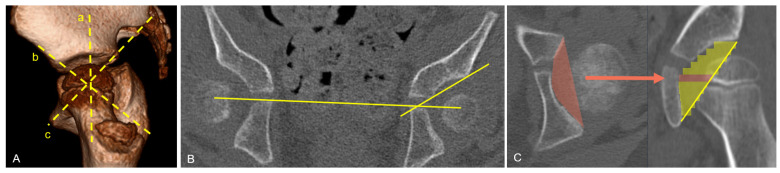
(A) Measurement of the superolateral index (a), anterosuperior index (b), and posterosuperior index (c). (B) Reformatted CT scan showing the anterosuperior index. (C) Calculation of the acetabular volume considering the slice thickness by integrating the area of the acetabulum [25].
